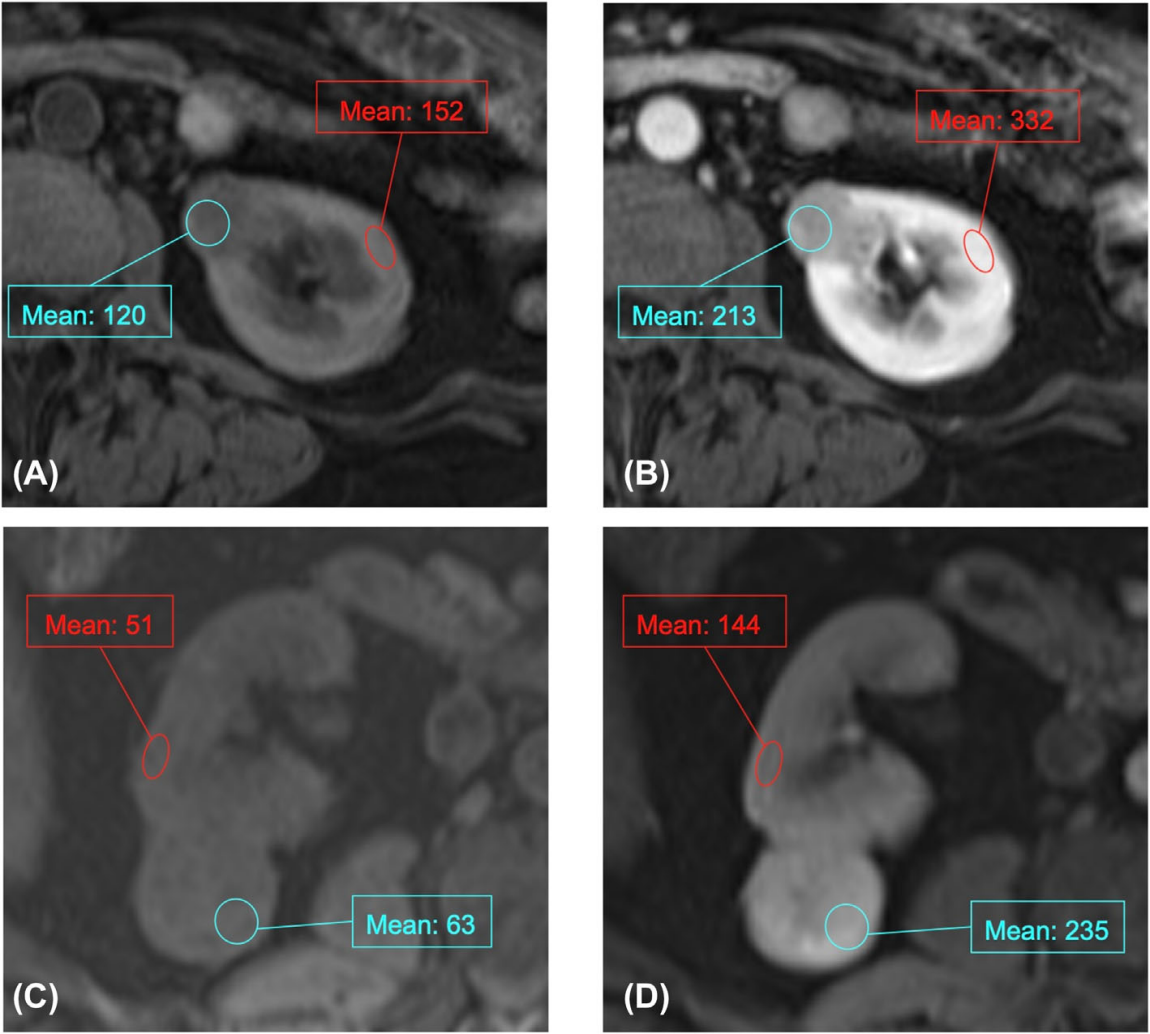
PEER measurement technique. T1‐weighted fat‐suppressed magnetic resonance images demonstrating ROI placement to measure signal intensities for PEER calculation within the tumour (blue circles) and within the renal cortex (red ovals) on (a) pre‐contrast image and (b) post‐contrast image in a patient with chRCC, and (c) pre‐contrast image and (d) post‐contrast image in a patient with oncocytoma. (a, b) PEER = (213–120)/(332–152) = 0.52. (c, d) PEER = (235–63)/(144–51) = 1.85.
